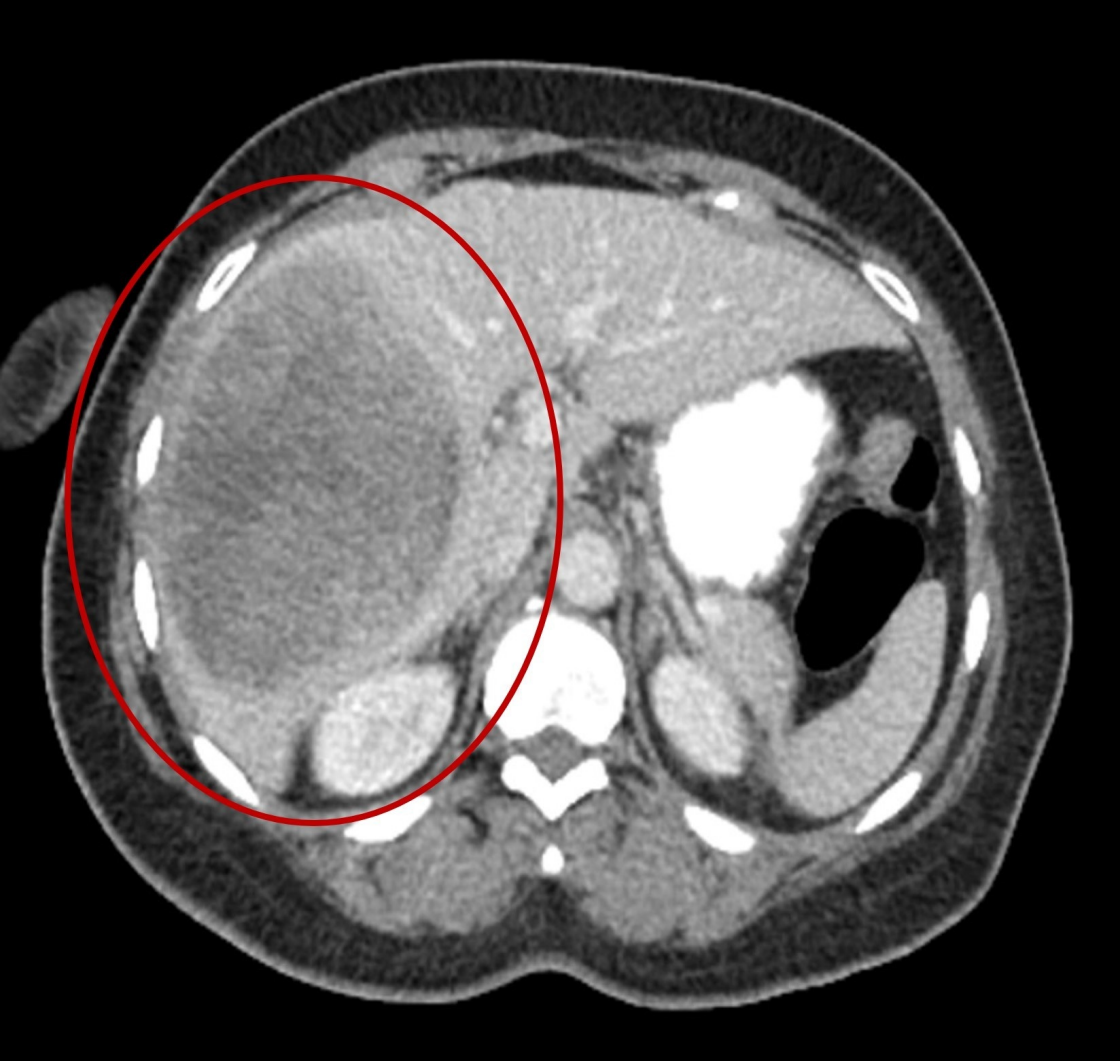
CT of the abdomen: 11 cm mass in the right lobe. CT: computed tomography
